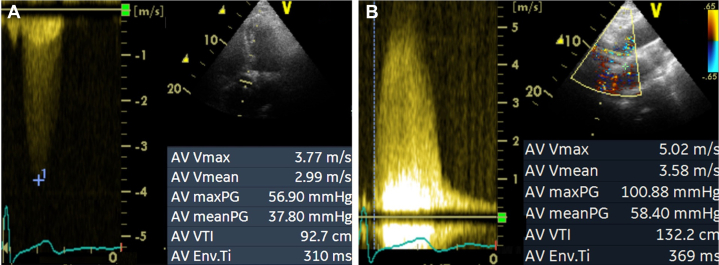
Severe Aortic Valve Stenosis The right parasternal approach identified a very severe stenosis (B) vs the apical view (A).
